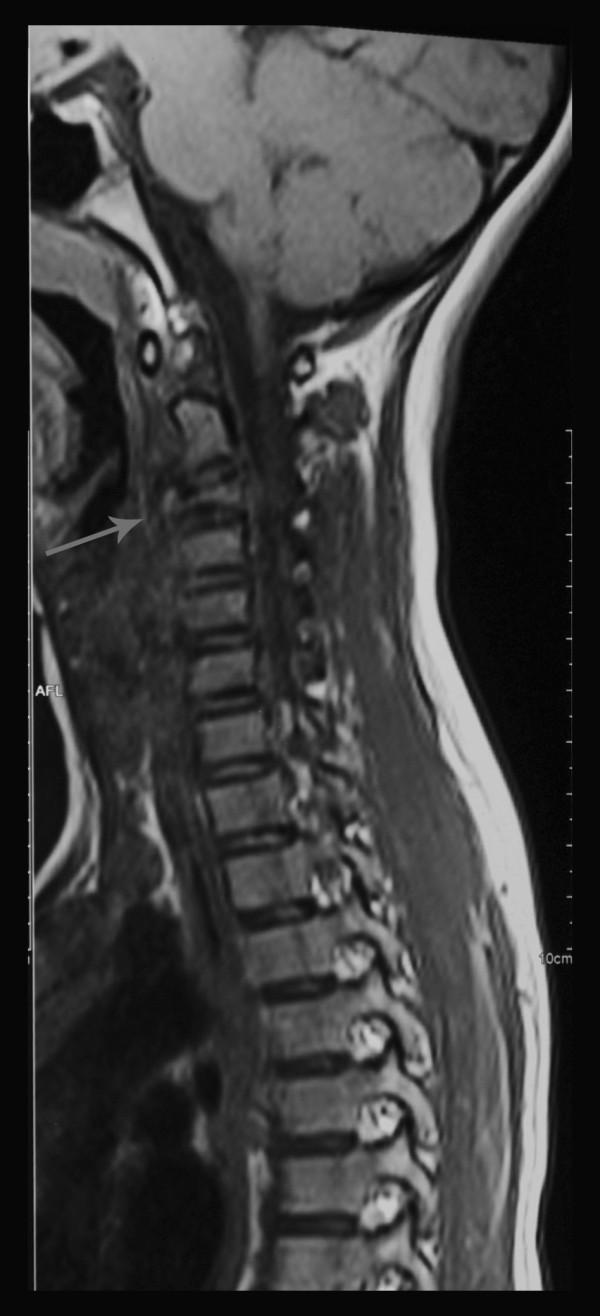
Magnetic resonance imaging showing loss of third cervical vertebra body height, asymmetrical prevertebral soft tissue thickening (cold-abscess) (arrow) and indentation of thecal sac without cord compression.
